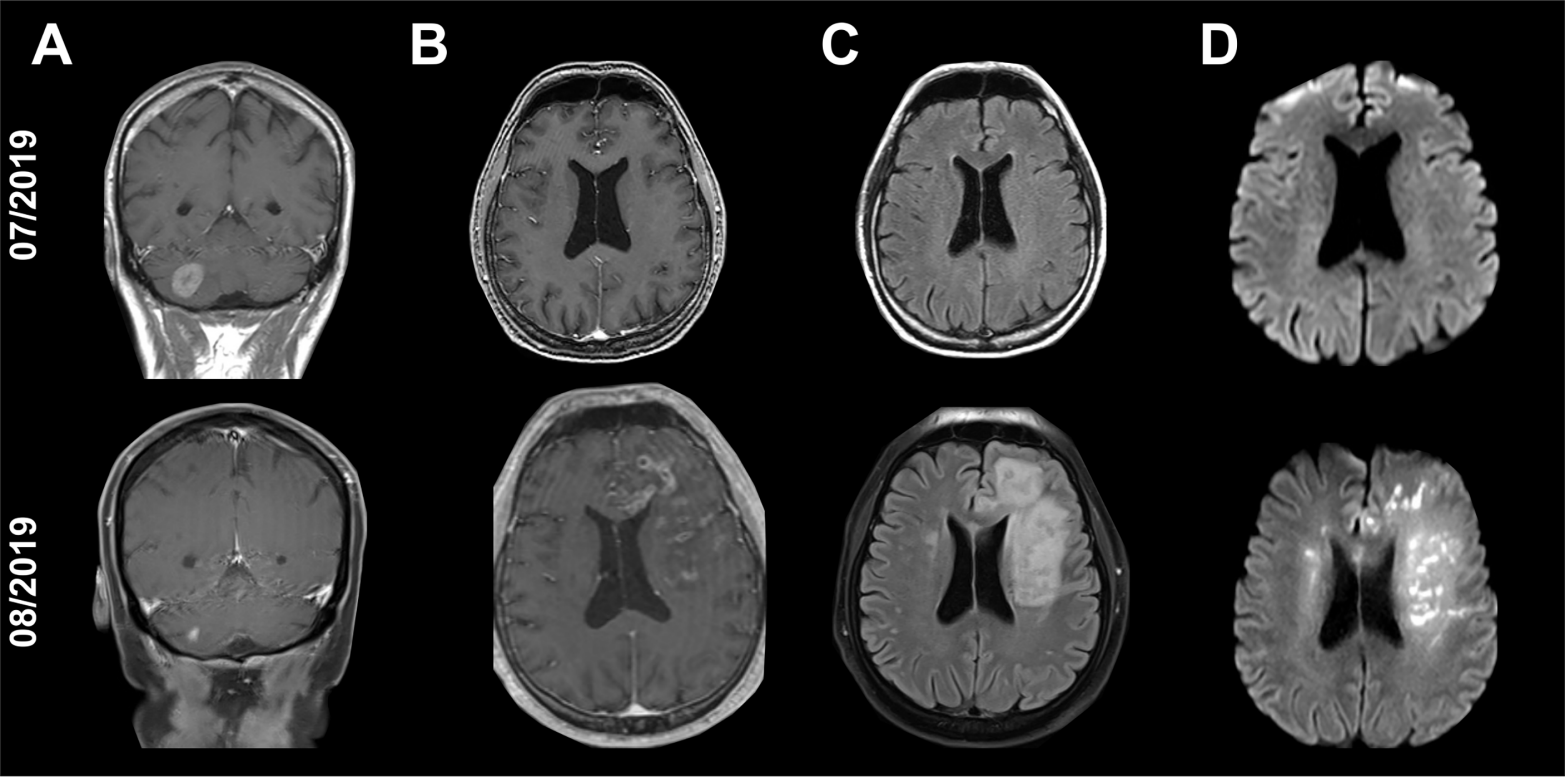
Cranial MRI scans of a 54-year-old patient with single, cerebellar metastasis of non-small-cell lung carcinoma. The MRI scan shows an excellent response of the cerebellar metastasis to the radiation therapy [(A): T1-weighted, contrast enhanced images]. At the same time, new tubular contrast enhancements with adjacent edema and diffusion restrictions have appeared in the left frontal lobe distant to the irradiated cerebellar metastasis [(B): T1-weighted, contrast enhanced images, (C): Fluid-attenuated inversion recovery (FLAIR), (D): Diffusion-weighted images (DWI, b1000)].
